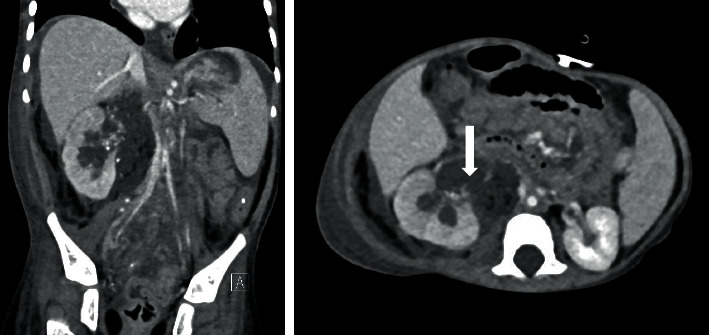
CT image showing rupture of the right ureter. A focal wall defect was noted at the posterior aspect of the right proximal ureter, just distal to the right ureteropelvic junction (arrow) with adjacent fluid surrounding the ureter.
